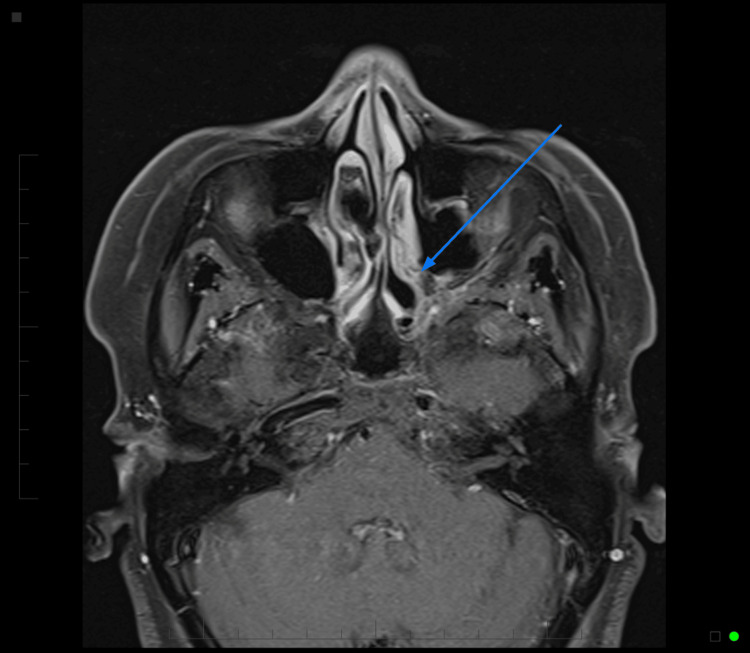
Axial post-gadolinium T1-weighted fat-saturated MRI following antifungal therapy demonstrating improvement with resolution of abnormal enhancement in the retromaxillary region and vidian canal. Residual soft tissue thickening within the left pterygopalatine fossa persists (arrow) MRI: magnetic resonance imaging
